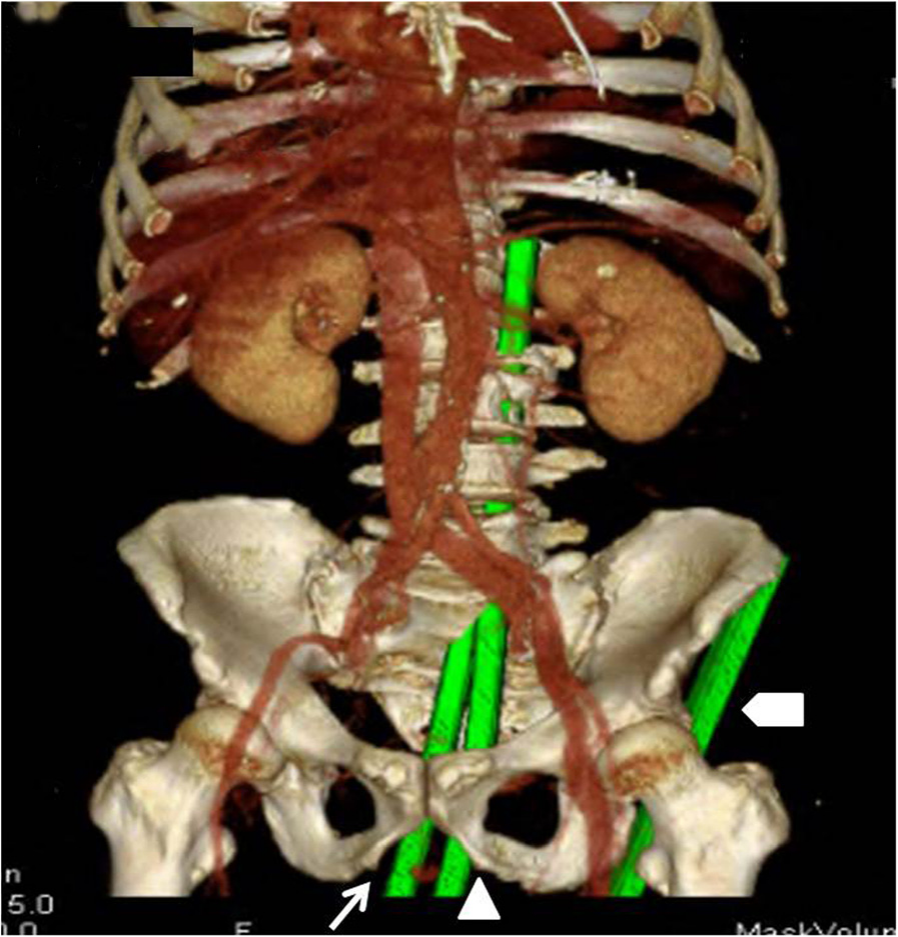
Computed tomography reconstruction showing the location of the three steel bars: bar A (⌂; left), bar B (∆; center), and bar C (↑; right). Bar A (left) pierced the left gluteal region and extended into the left anterior superior iliac spine. Bar B (center) pierced the right gluteal region, passed through the rectum, continued into the spinal canal from the sacral bone, and extended into the spinal canal at level L4. Bar C (right) pierced the perineal region, passed through the rectum, continued into the spinal canal from the sacral bone, exited the spinal canal at the left side of L3, and extended into the posterosuperior side of the left kidney.
